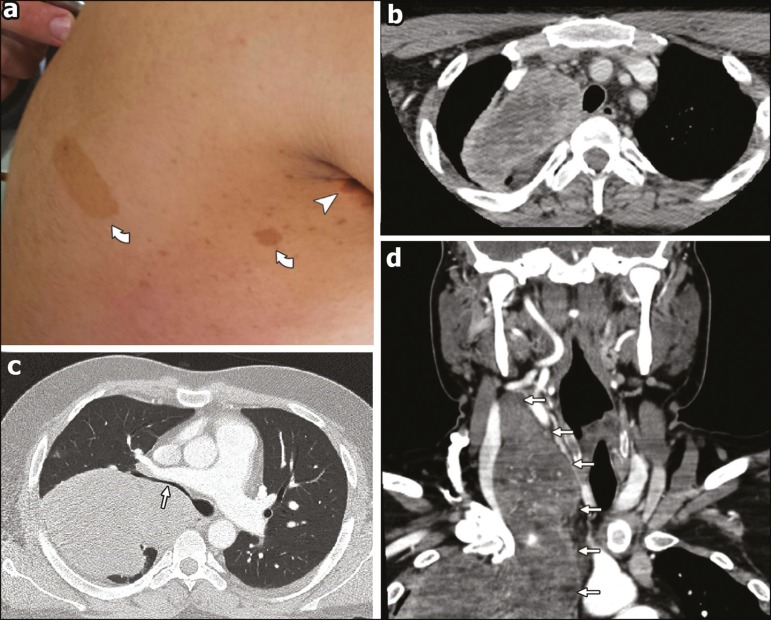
Findings on physical examination and CT. a:
